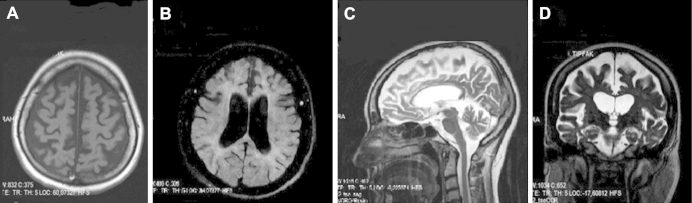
Magnetic resonance imaging of the proband. (A) Transverse T1-weighted image shows atrophy of the frontoparietal cortices. (B) Transverse fluid-attenuated inversion recovery image demonstrates mild but diffuse hyperintense appearance of the white matter, mainly located in the neighborhood of the ventricle horns. (C) In sagittal T2-weighted image, there is marked thinning of the entire corpus callosum. (D) Coronal T2-weighted image reveals enlargement of the lateral and third ventricles with atrophy of the bilateral caudate nuclei.
